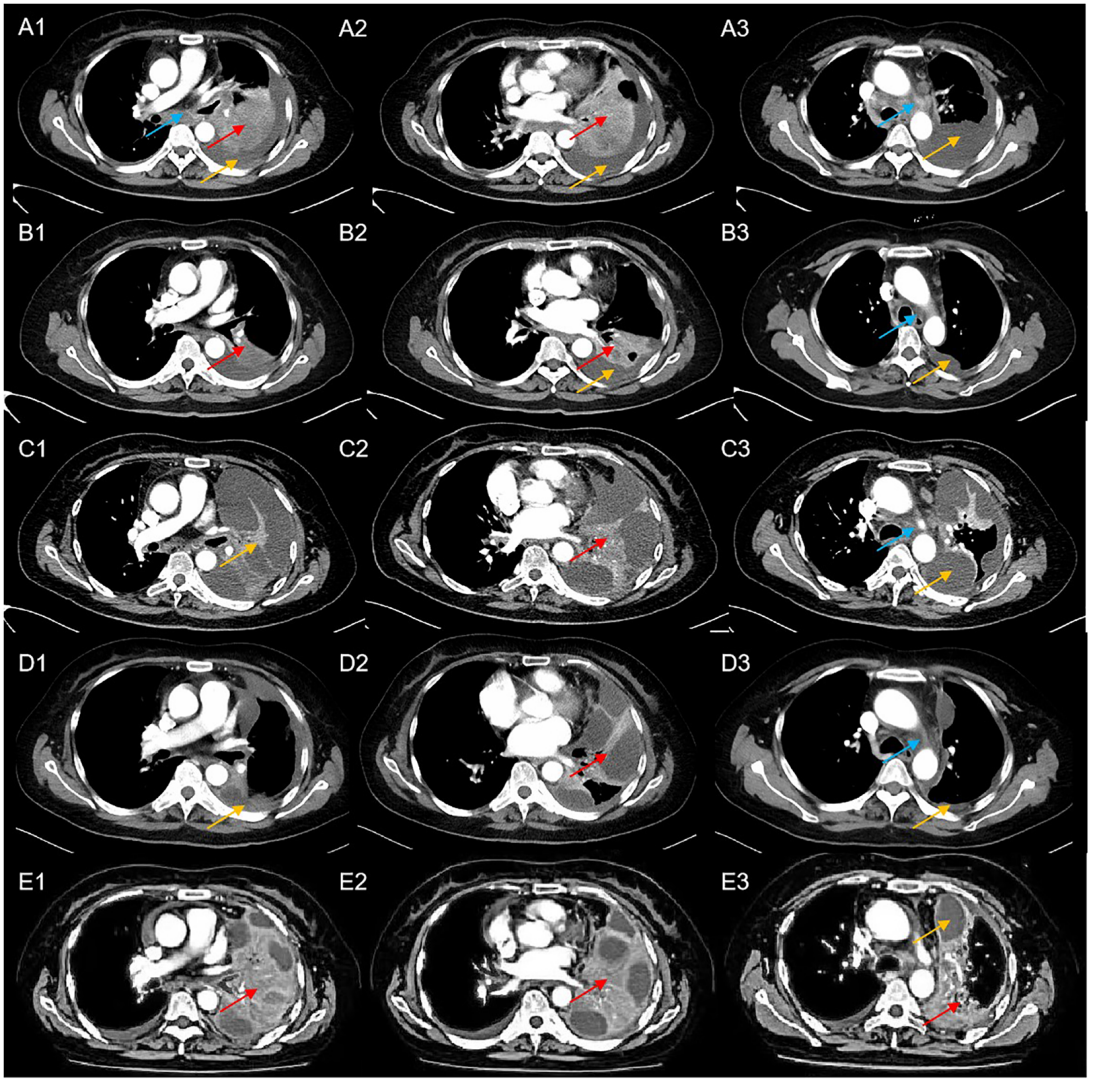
Computed tomography scans of the patient at a particular course of disease (blue arrows indicated mediastinal lymphadenopathy, red arrows indicate the location of the tumor, yellow arrows indicated left pleural effusion); (A1-A3) CT scans at baseline; (B1-B3) CT scans after 1 month of treatment with alectinib revealed a dramatic reduction in tumor size and pleural effusion; (C1-C3) CT scans after three months of treatment with alectinib showed disease progression; (D1-D3) CT scans after one month of treatment with ensartinib revealed partial response of the primary tumor and pleural effusion; (E1-E3) CT scans after two months of treatment with ensartinib revealed disease progression.
